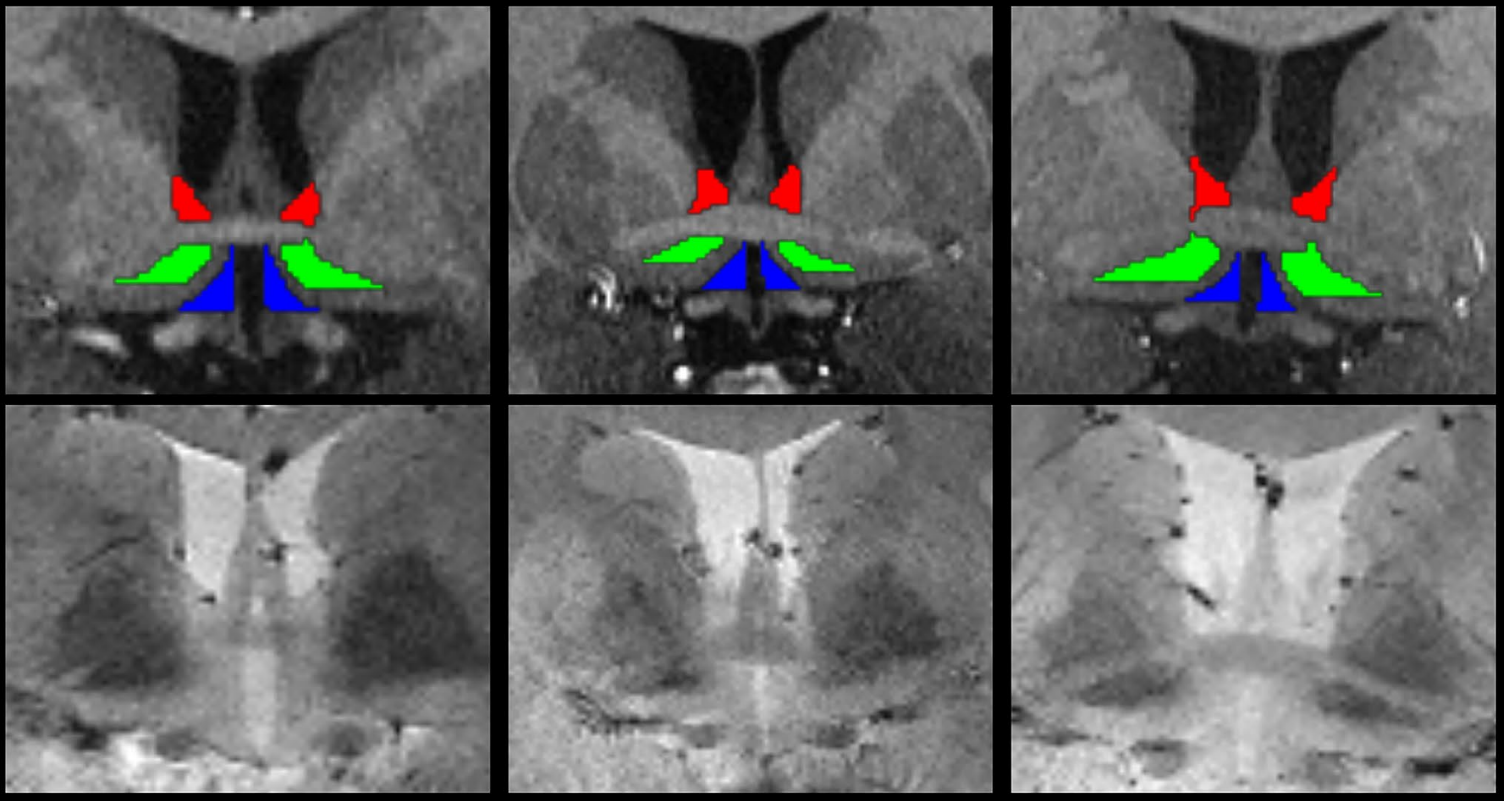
Manual segmentations of the dorsal (red) and ventral (green) bed nucleus of the stria terminalis and paraventricular nucleus of the hypothalamus (blue) in three representative native space T1-weighted MPRAGE images (Top) with respective high resolution gradient echo (2D GRE) (Bottom), demonstrating how each segmentation is uniquely influenced by individual neuroanatomical differences in boundary-driving structures
